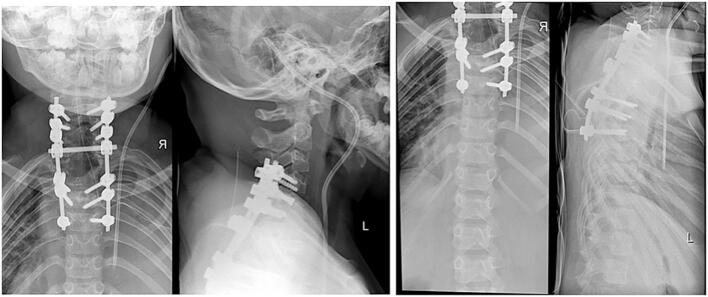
Postoperative X-ray of the cervical and thoracal, AP and lateral.
